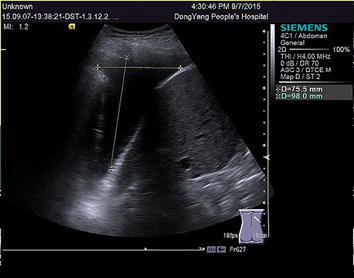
Ultrasound on September 7 2015 showed that the diameter of anteroposterior and vertical respectively was 98 and 76 mm in the *right*, and 33 and 71 mm in the *left* (1 month later)
